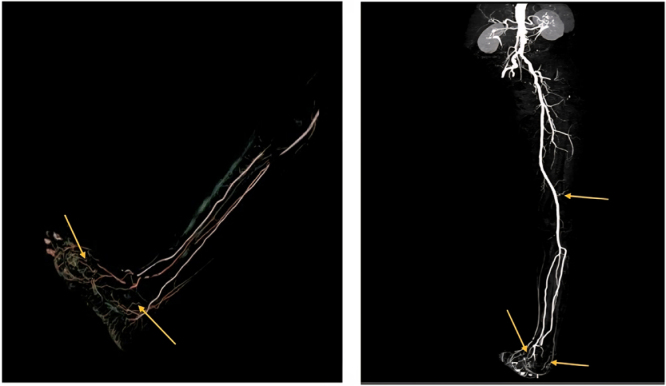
Computed tomography angiography with 3D reconstruction of the left lower limb demonstrating distal collateral vessel formation with relative sparing of the proximal arteries and distal arterial refilling through prominent tortuous corkscrew collateral vessels around the foot (arrows), consistent with microvascular involvement. An angiographic pattern characteristic of thromboangiitis obliterans in the appropriate clinical context.
